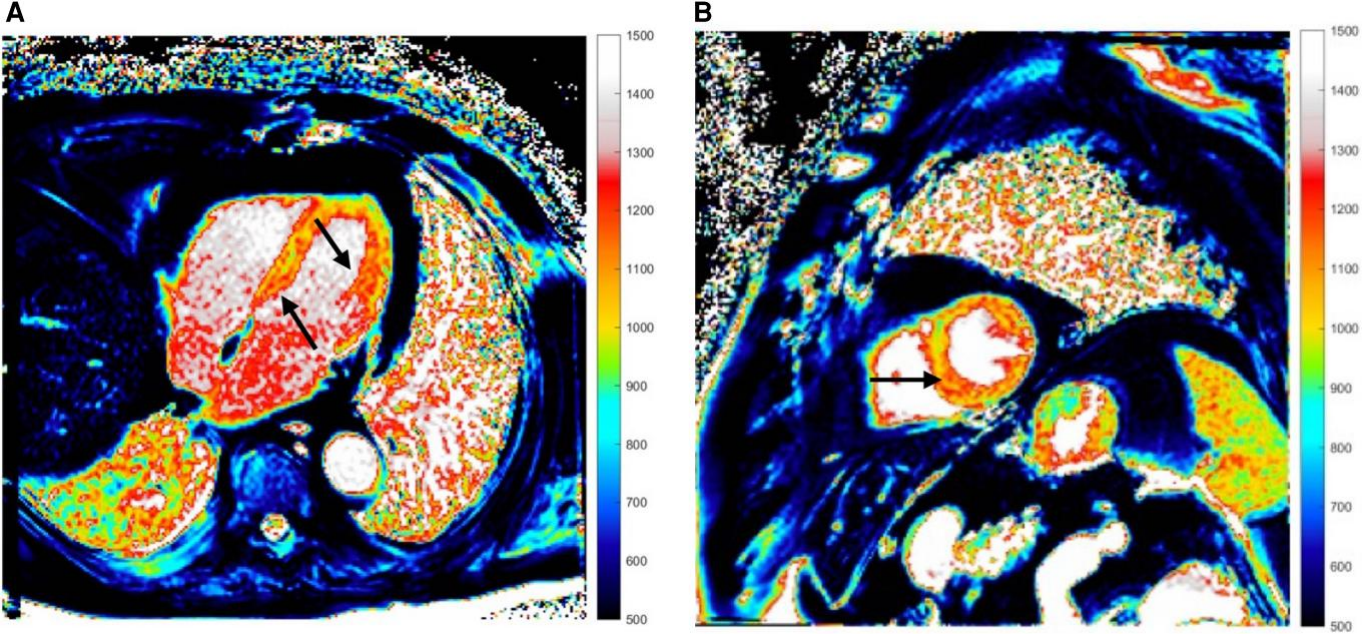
Cardiac MRI native T1 long and midventricular short axis view during the initial hospitalization. Normal myocardial native T1 at 1.5T is ∼965–1025 ms. Diffusely increased values, highlighted by black arrows, indicated myocardial oedema and inflammation (MRI, magnetic resonance imaging; ms, milliseconds).
